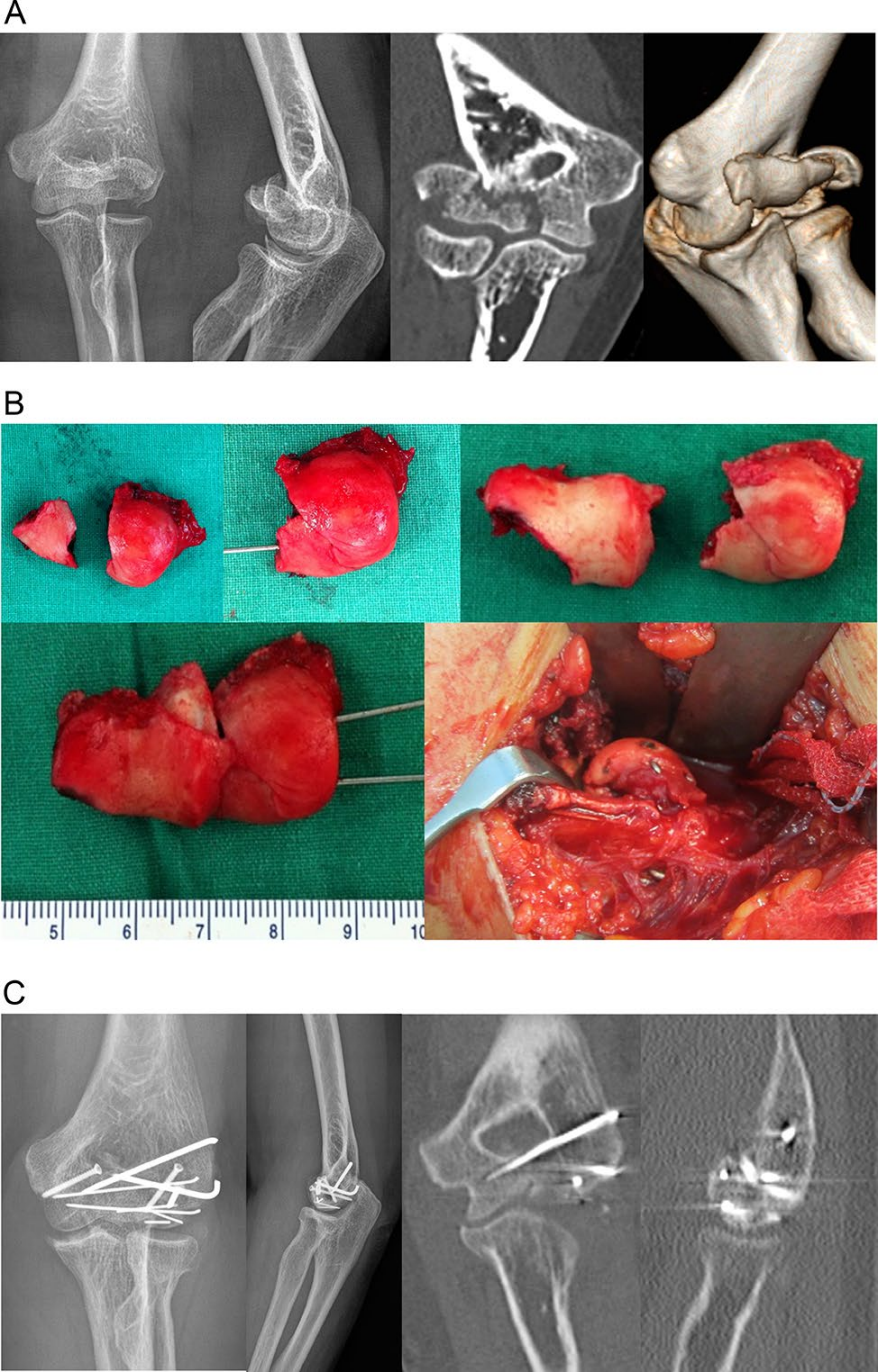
(A) Preoperative x-ray and CT scans of Dubberley type IIIA fracture. (B) ‘on-table’ reconstruction using subchondral K-wires. (C) postoperative x-ray and CT scan showing union after 1 year
